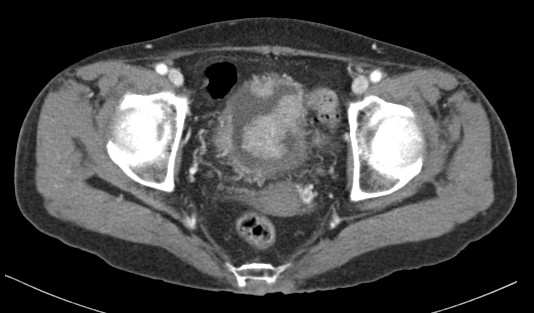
Preoperative computed tomography scan demonstrated multiple enhancing masses in the urinary bladder.
